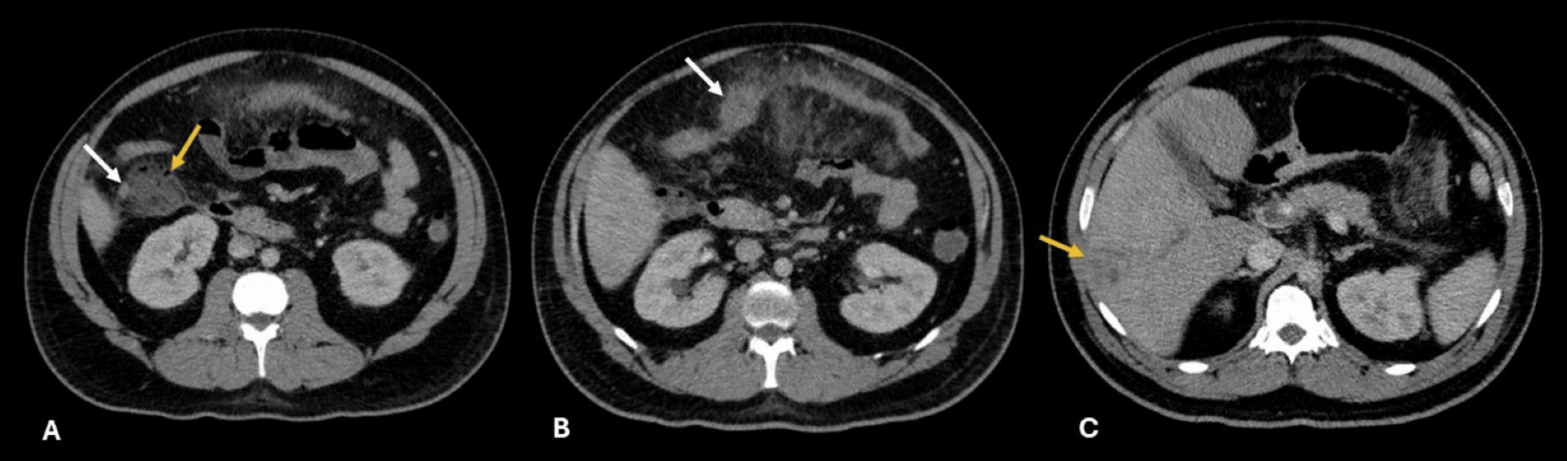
Axial, portal-phase contrast-enhanced abdominal CT A) Cecal appendicitis with wall hyperenhancement (white arrow) and adjacent abscess (yellow arrow). B) Transverse colon wall thickening with mild hypodensity (white arrow), mesenteric fat stranding, and fluid. C) Confluent microabscesses in the right hepatic lobe (yellow arrow).
